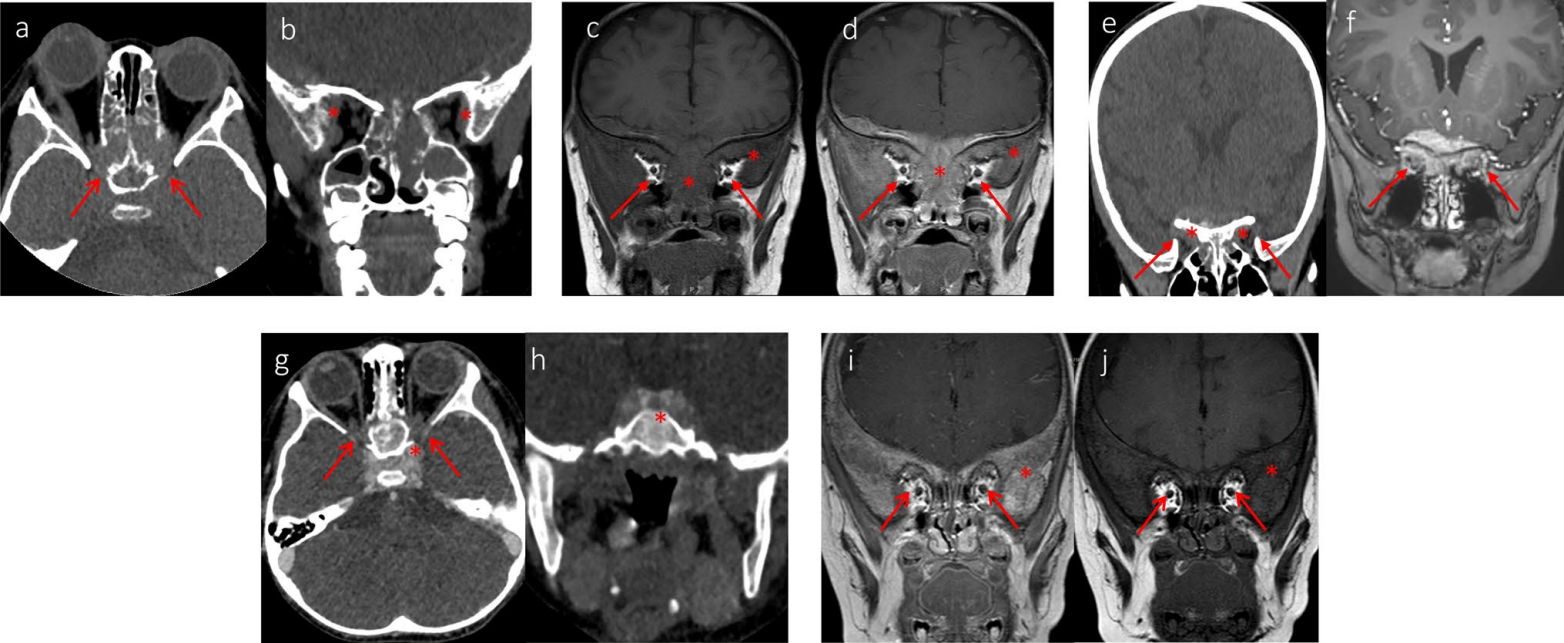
Head imaging of five children. a, b CT appearance of the head metastatic tumor in Case No. 1; c, d MRI appearance of the head metastatic tumor in Case No. 2; e CT appearance and f MRI appearance of the head metastatic tumor in Case No. 3; g, h CT appearance of the head metastatic tumor in Case No. 4; i, j MRI appearance of the head metastatic tumor in Case No. 5. Metastatic tumor lesion involving the optic foramen with compression of the optic nerve (arrow) and bone metastatic lesion with bone destruction and soft tissue formation (asterisk)
